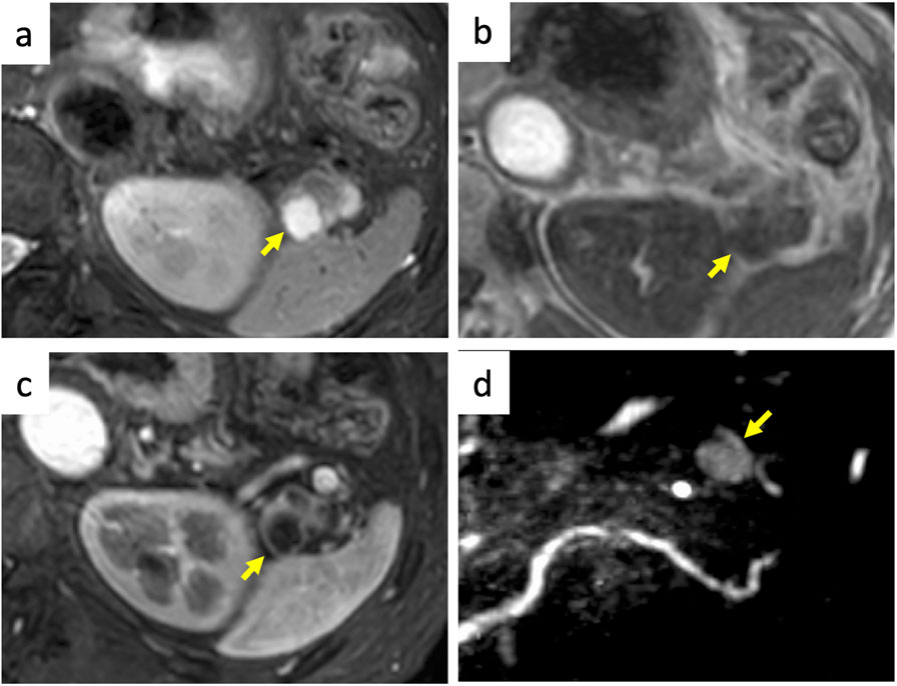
Magnetic resonance images. a Cystic components show varying intensities on T2-weighted images (yellow arrow). b Cystic components exhibit low intensity on T1-weighted images (yellow arrow). c Cystic wall is enhanced on contrast-enhanced magnetic resonance imaging (yellow arrow). d Magnetic resonance cholangiopancreatography showing that there is no indication to suggest a connection between the pancreatic lesion and the main pancreatic duct
